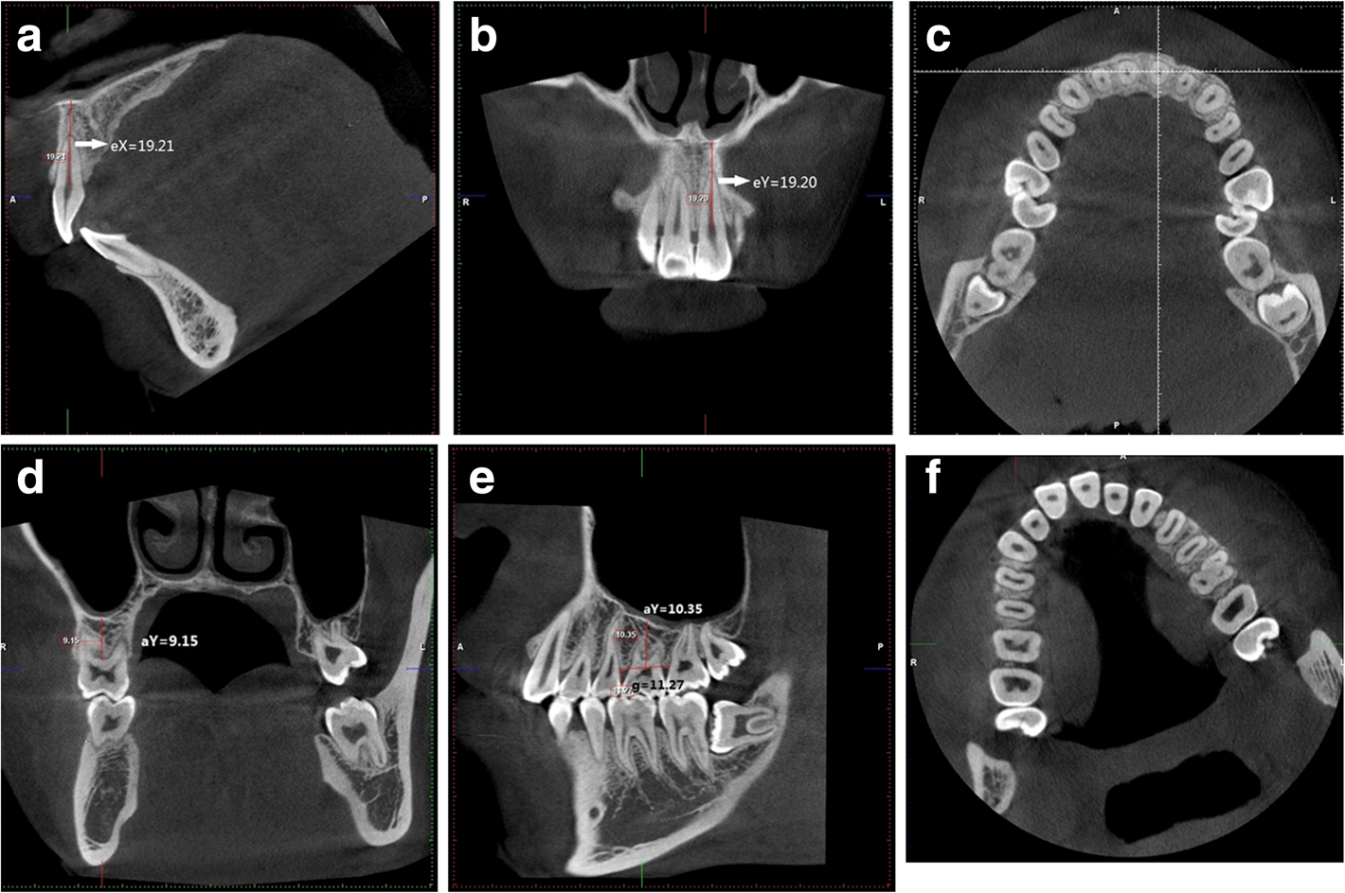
CBCT images showing the vertical measurement on X a, Y b and Z c planes and horizontal measurement d-f
